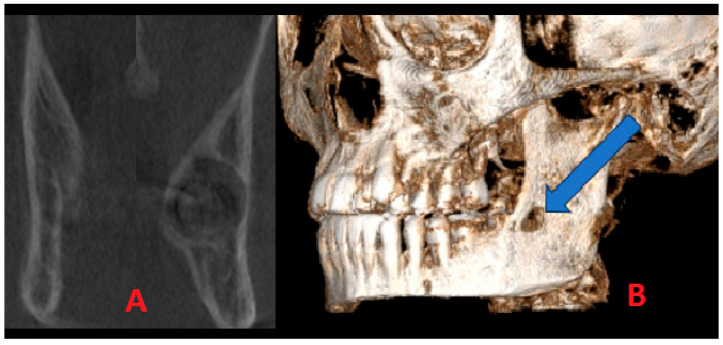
The CBCT visualization of the lesion: (A) bone asymmetry; (B) extracortical spread marked with blue arrow.
